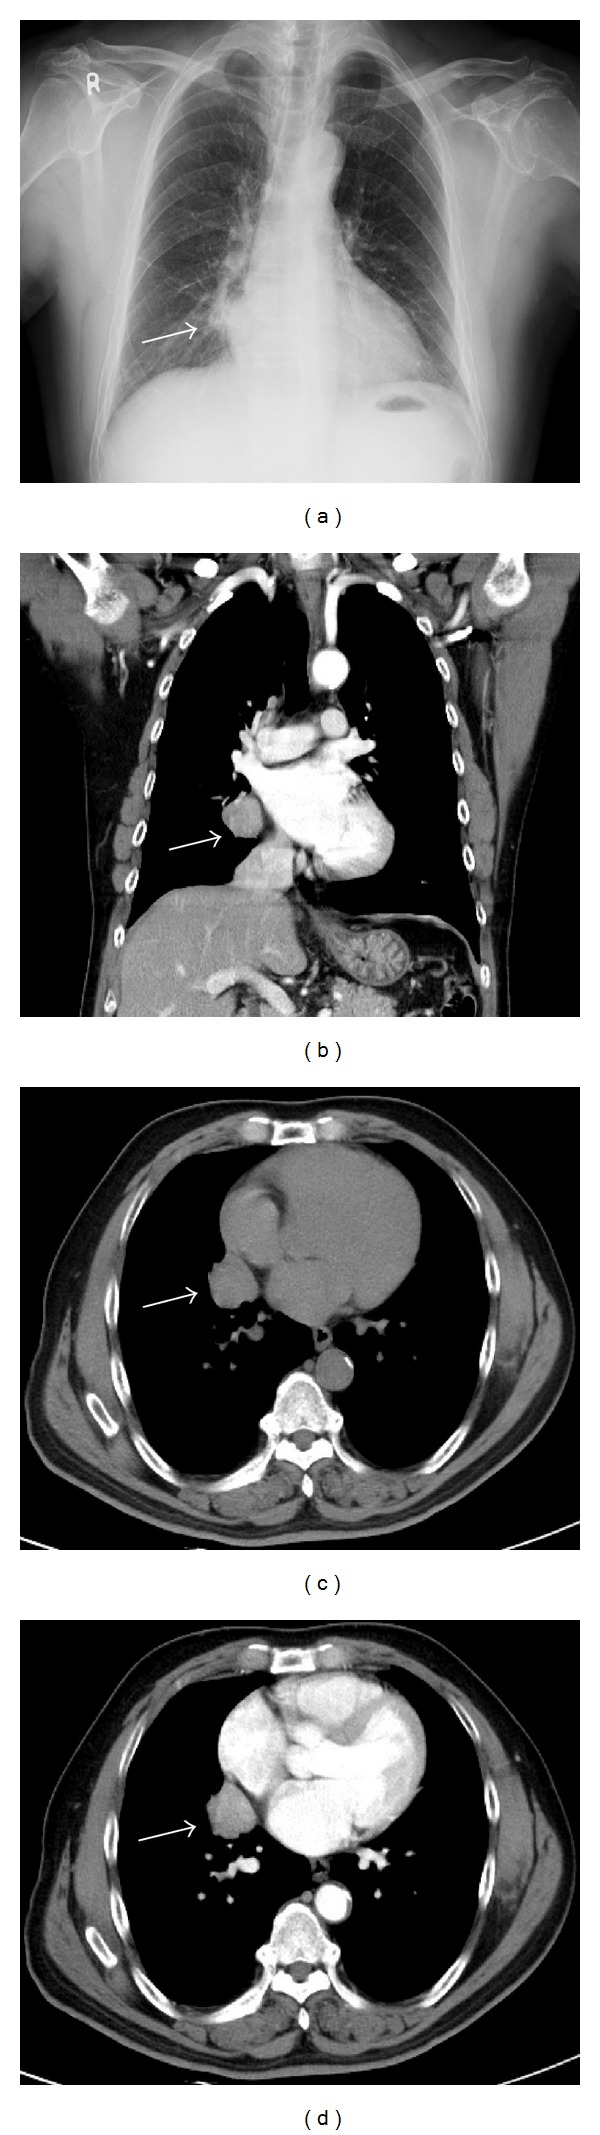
(a) Chest X-ray showed a mass lesion in the right paramediastinal region (arrow); (c) Noncontrast-enhanced CT scan: an isodensity lobulated mass lesion in the right middle lobe of lung; ((b) and (d)) Chest CT scan showed a 30 × 29 mm heterogenously enhanced mass lesion with well-defined lobulated margin in the right middle lobe of lung, abutting the mediastinum.
